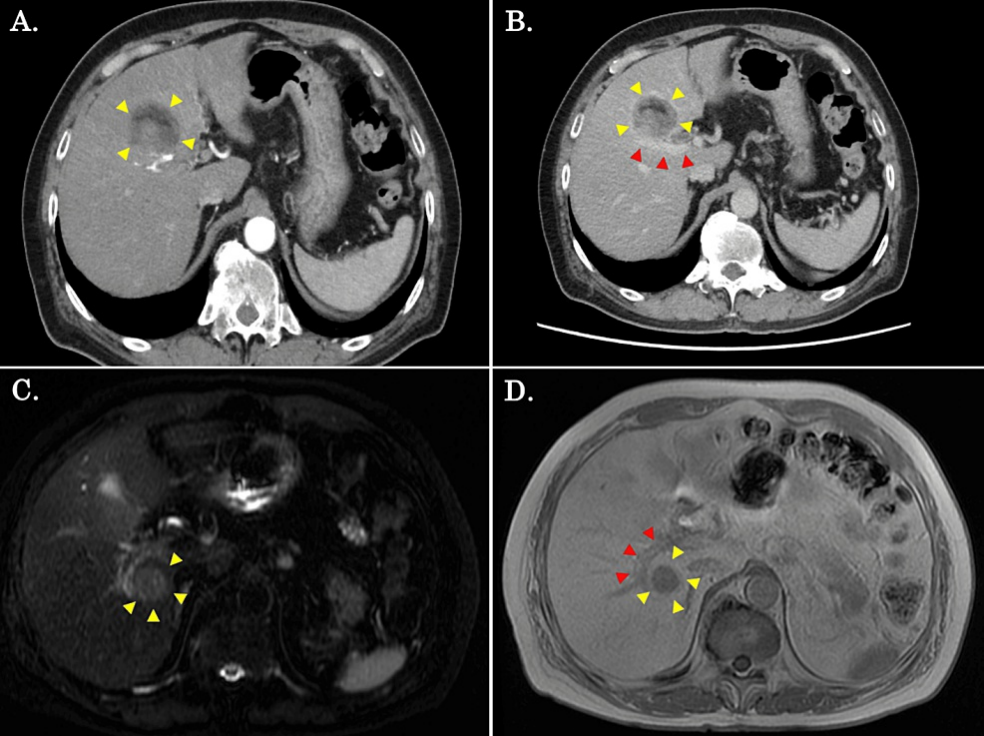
CT and MRI findings prior to the biological therapy. (A, B) An enhanced CT scan showed a 40 mm-sized S4/S5 tumor (yellow arrows), with broad contact with the Glissonean pedicle of the right anterior section (red arrows). (C, D) An MRI showed a 27 mm-sized S7 tumor (yellow arrows) adjacent to the right posterior Glisson (red arrows).
